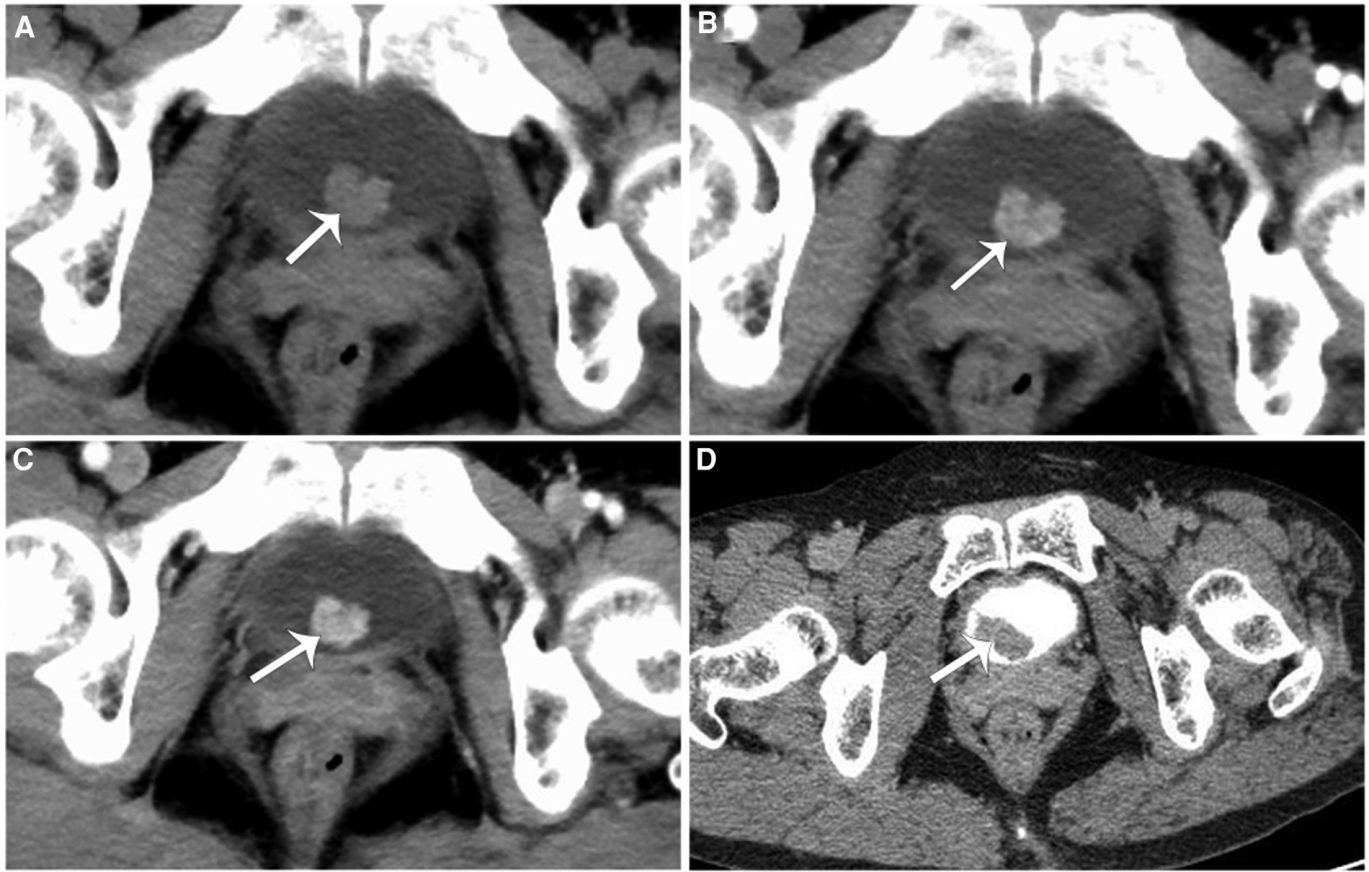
(A–C) A cauliflower-shaped soft tissue density nodular shadow (white arrow) was seen in the right posterior lower wall of the bladder, measuring approximately 12 mm × 11 mm × 11 mm, with a wide base attached to the bladder wall and an irregular morphology. (D) Enhancement scan, computed tomography urography (CTU) showed a soft tissue density filling defect in the right posterior lower wall of the bladder.
